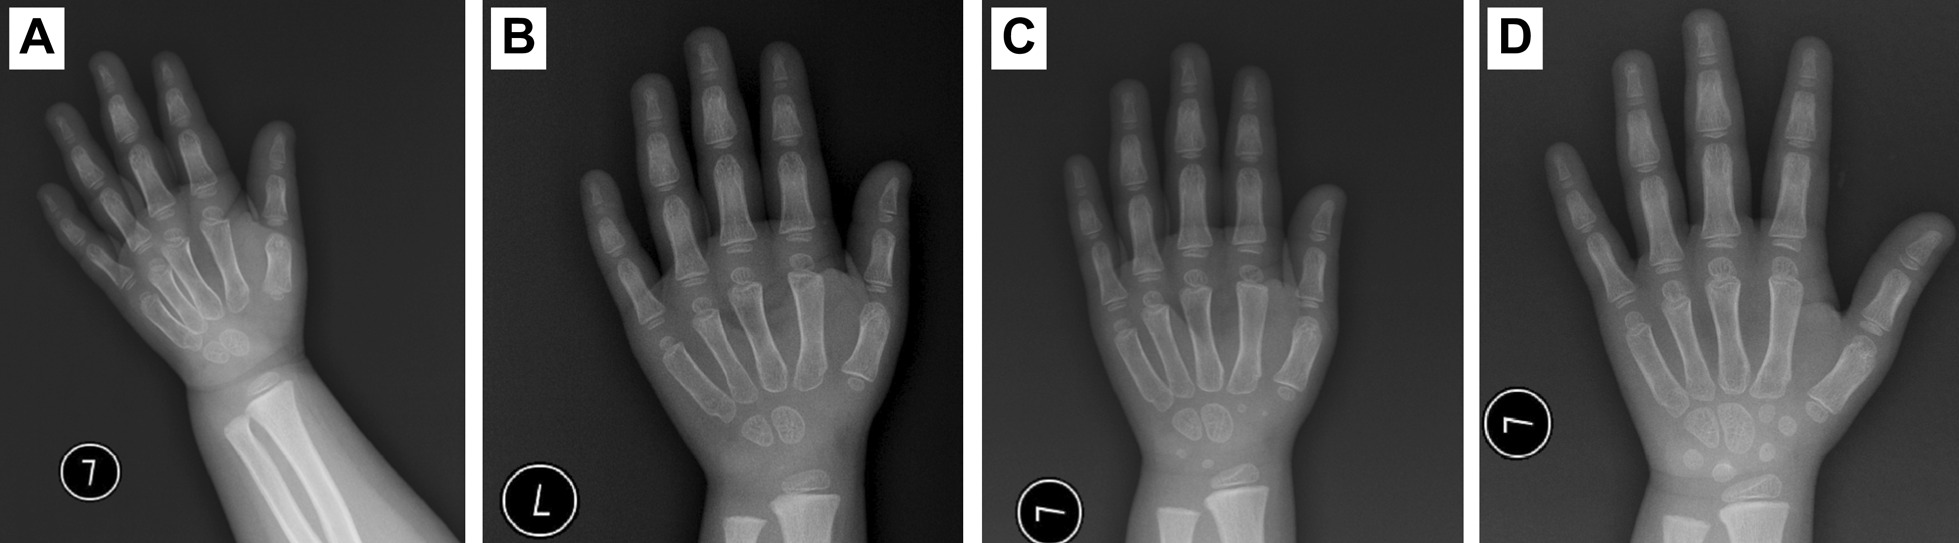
Bone age radiograph in the course of GH treatment. A CA: 4–3/12 years; BA: 2.5 years. B CA: 4–9/12 years; BA: 3 years. C CA: 5–4/12 years; BA: 4 years. D CA: 6–4/12 years; BA: 5 years
